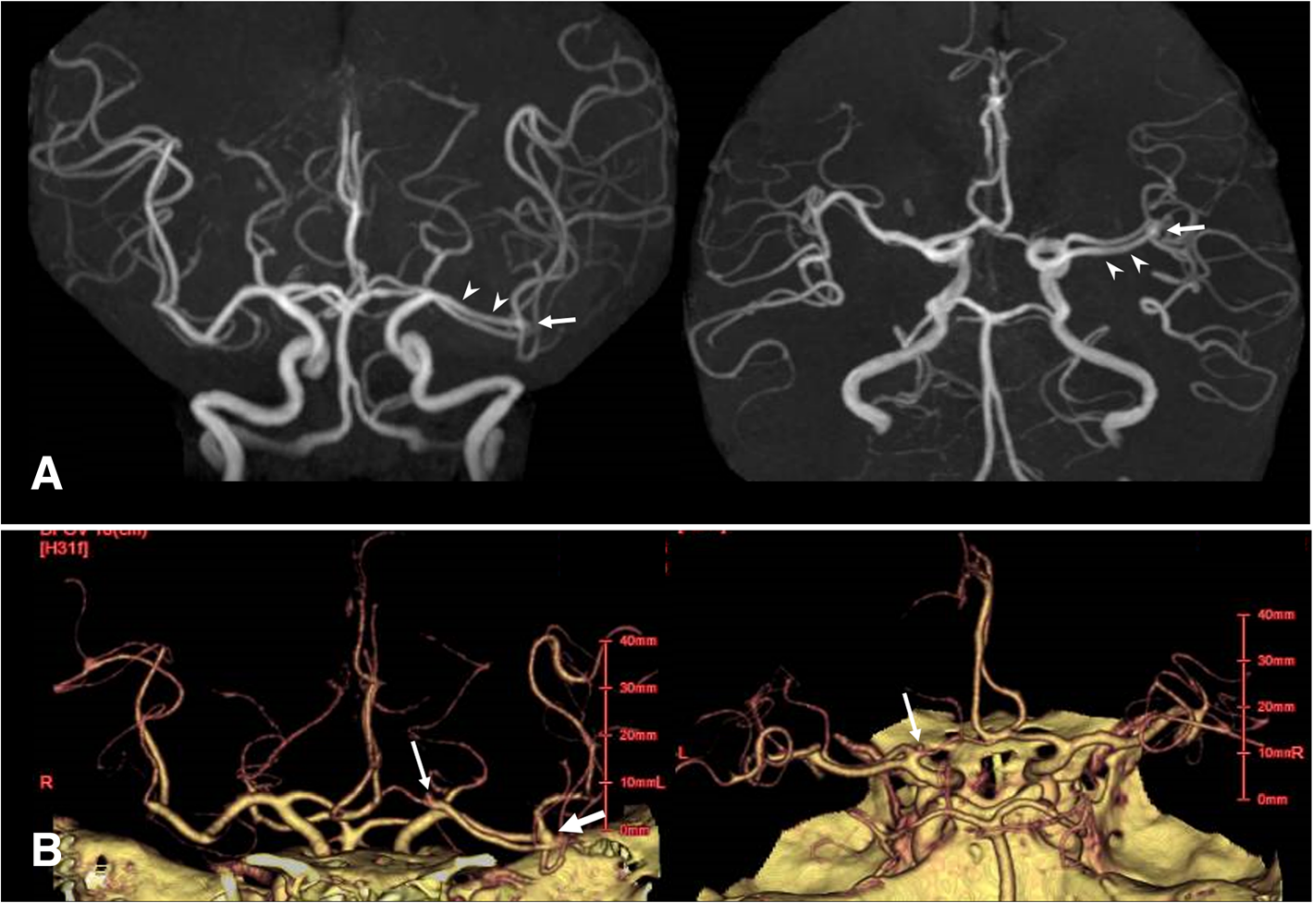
a Magnetic resonance angiography showing duplicated middle cerebral artery (*arrowheads*) on the left and an aneurysm at the M1/M2 junction of duplicated middle cerebral artery (*arrow*). b Three-dimensional computed tomographic angiography also demonstrating the left duplicated middle cerebral artery and aneurysms at the M1/M2 junction of duplicated middle cerebral artery (*arrow*) and internal carotid artery top (*small arrow*)
